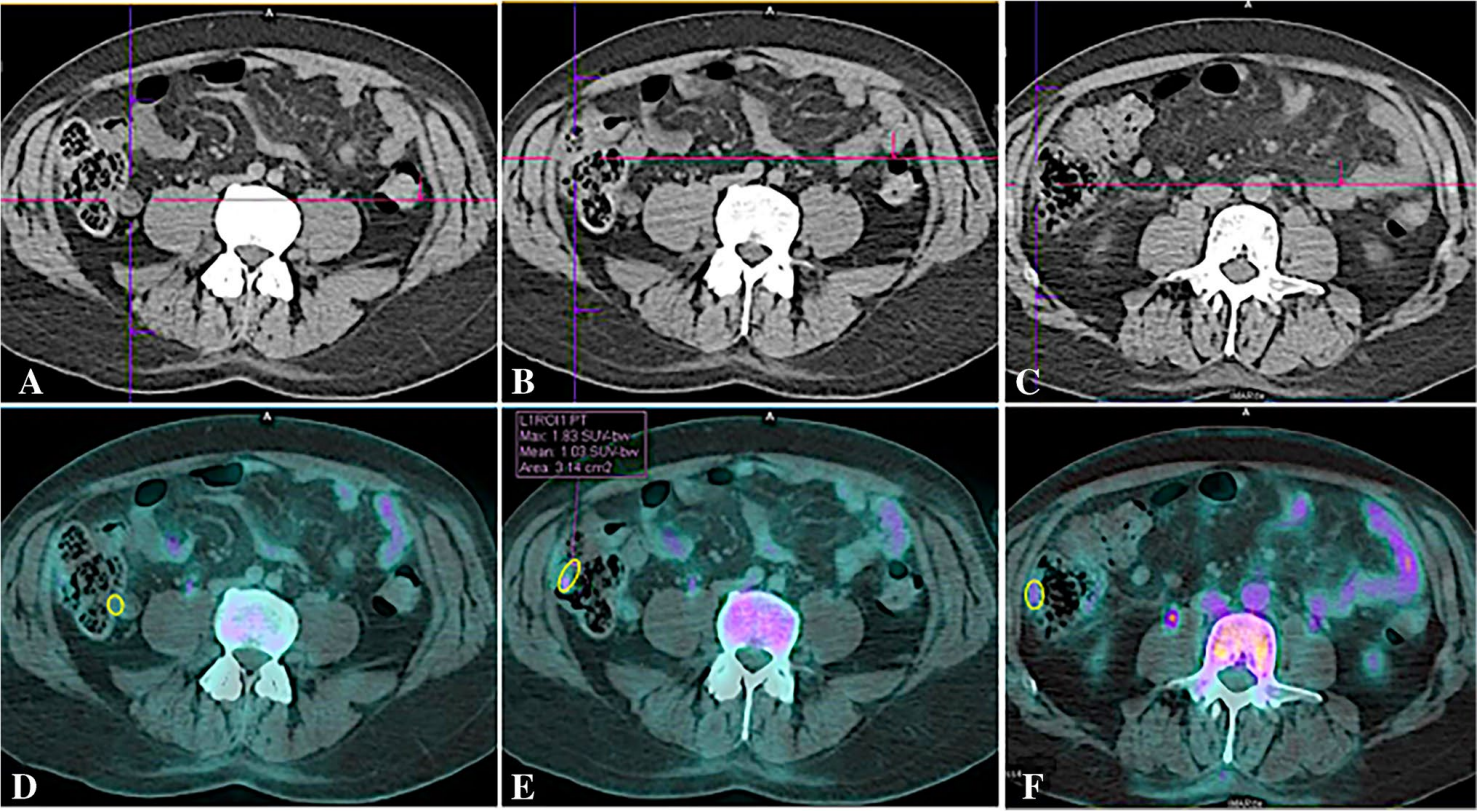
Axial PET/CT images in a patient at the time of diagnosis, showing how we measured the activity in the bowel wall. Regions with adequate thickened walls were selected by the radiologist on CT slices (A–C), and then circular ROIs were drawn over the corresponding PET/CT images (D–F). The software provides the measurement of SUV_max_, SUV_mean_, and total ROI area. Images A and D show the ROI in the terminal ileum; B and C the ROI in the caecum, and C and F the ROI in the ascending colon of a newly diagnosed patient
